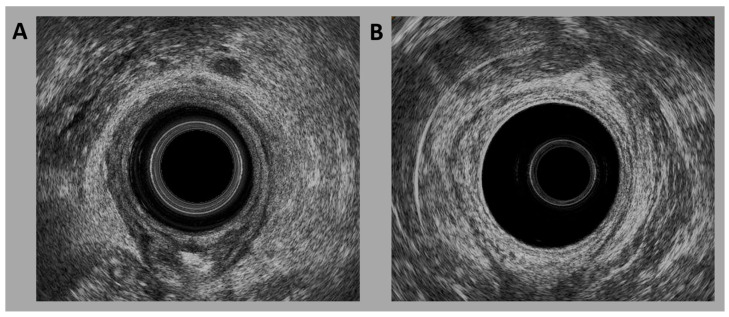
Transrectal ultrasound images: (A) rectal cancer and (B) normal rectum.
